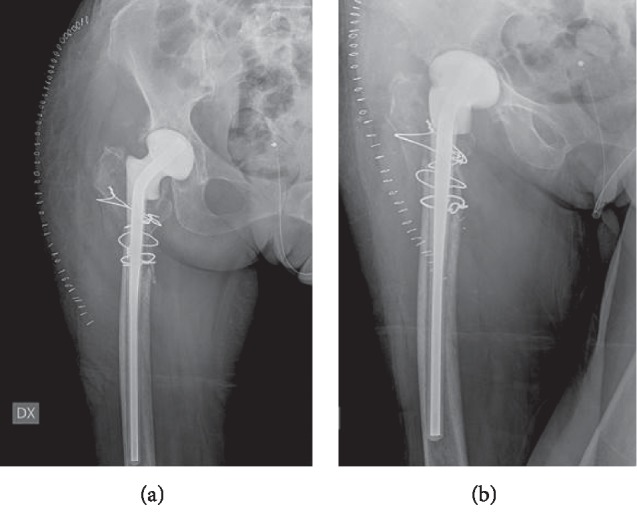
Hip radiography: anteroposterior and oblique views after prosthesis explant and spacer positioning.
